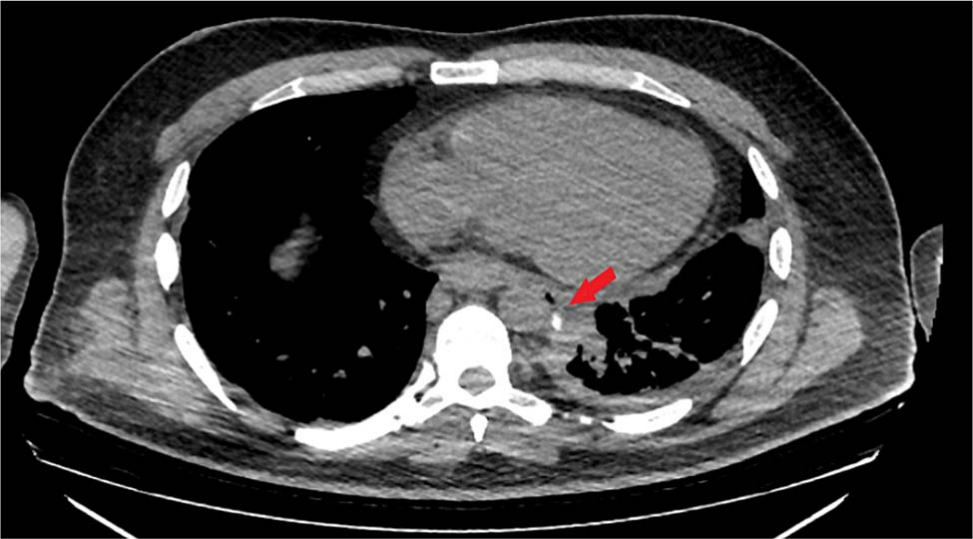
Chest CT scan with oral contrast showing significant reduction in the amount of leaked contrast (small rim remaining) with mild decrease in the amount of left pleural effusion and associated collapse consolidation as well as pneumomediastinum.
